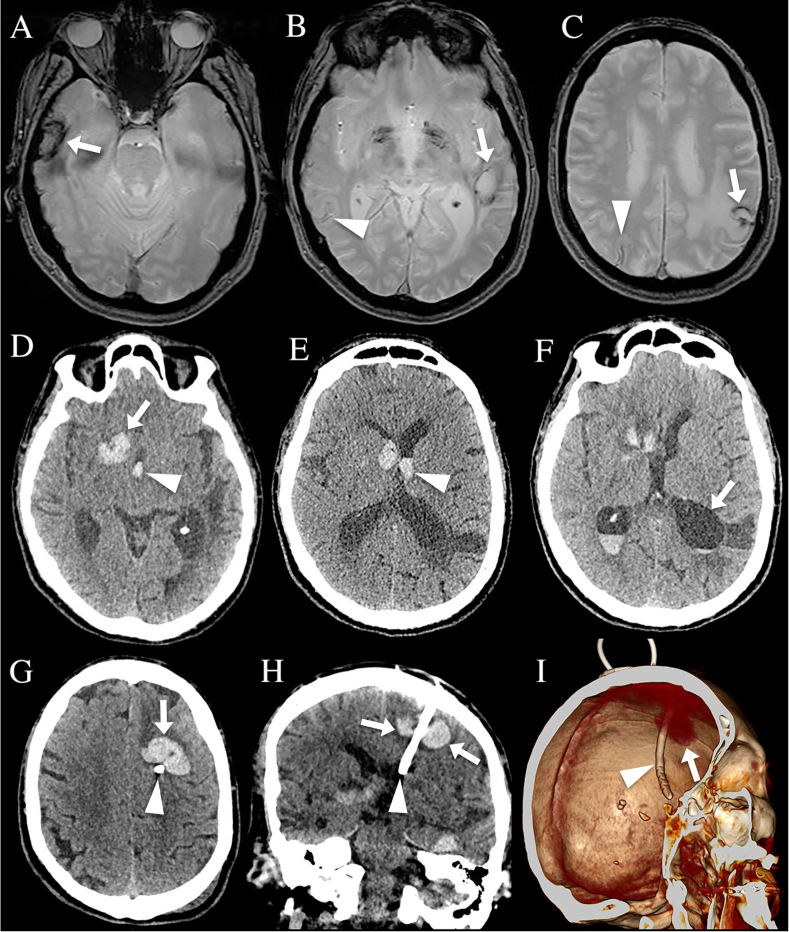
CAA patient 2 showing multiple chronic ICH (A-C, arrows) and cortical superficial siderosis (B and C, arrowheads) on MRI, four months before recurrence of acute ICH. Acute right frontal lobar subcortical ICH (D, arrow) associated with intraventricular hemorrhage extension (D and E, arrowheads) can be observed on CT. After three days, the patient’s state worsened and CT showed occurrence of hydrocephalus (F). A left frontal intraventricular drain was inserted. Postoperative CT showed ICH (arrows) surrounding the intraventricular drain (arrowhead) an axial (G) and coronal (H) CT images and on 3D multiplanar reconstruction images (I).
